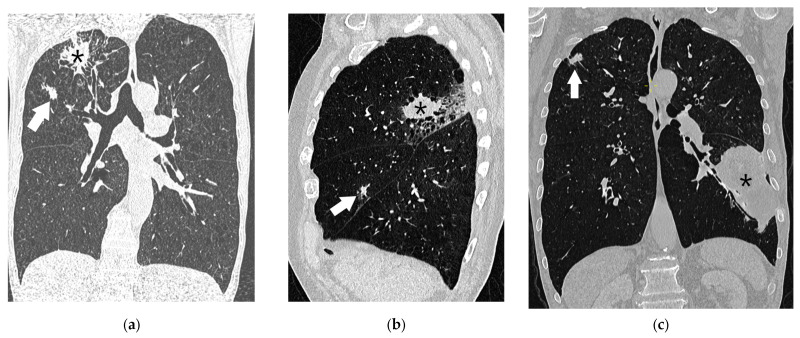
Additional lung nodules in three different patients, lung window setting (width 1500, level −600): A coronal reformatted chest CT image (a) showing a primary tumor (asterisk) and a metastatic lesion (arrow) in the upper-right lobe, corresponding to T3. A sagittal reformatted CT image (b) showing a primary tumor (asterisk) in the upper-right lobe and a metastatic lesion in the middle lobe (arrow), corresponding to T4. A coronal reformatted CT image (c) showing a primary tumor (asterisk) in the lower-left lobe and a metastatic lesion (arrow) in the upper-right lobe, corresponding to M1a.
